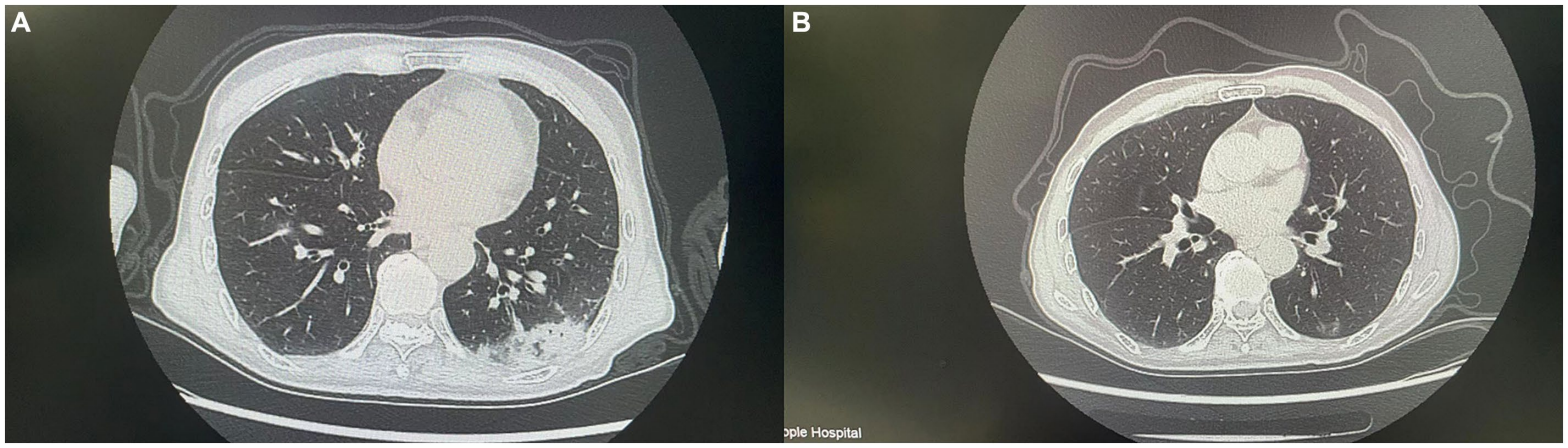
This figure shows CT images of the patient before and after antibiotic treatment. (A) (Before treatment) shows increased density in multiple areas of the lungs. (B) Shows a significant reduction in the inflammatory area and clearer lung structure.
